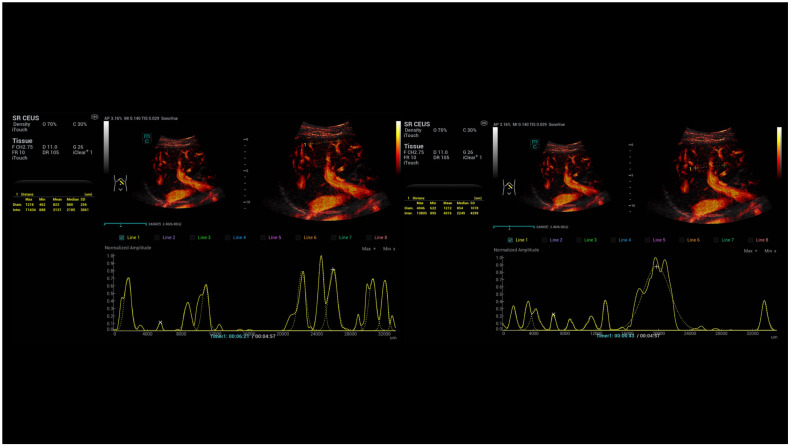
Diameter measurements of capillary vascalary structures near the liver capsular structure and in the center of the liver using a machine integradet SR CEUS quantification software. Independent evaluation by the stored dynamic SR CEUS cine loops over 5 s with manually adapted area’s near the capsular structures in comparison to the centre with peripheral capillary dilation in a case of Osler’s disease. CEUS in arterial phase after bolus injection of 2.0 mL ultrasound contrast agent (SonoVue) using a convex multifrequency probe (1–7 MHz).
